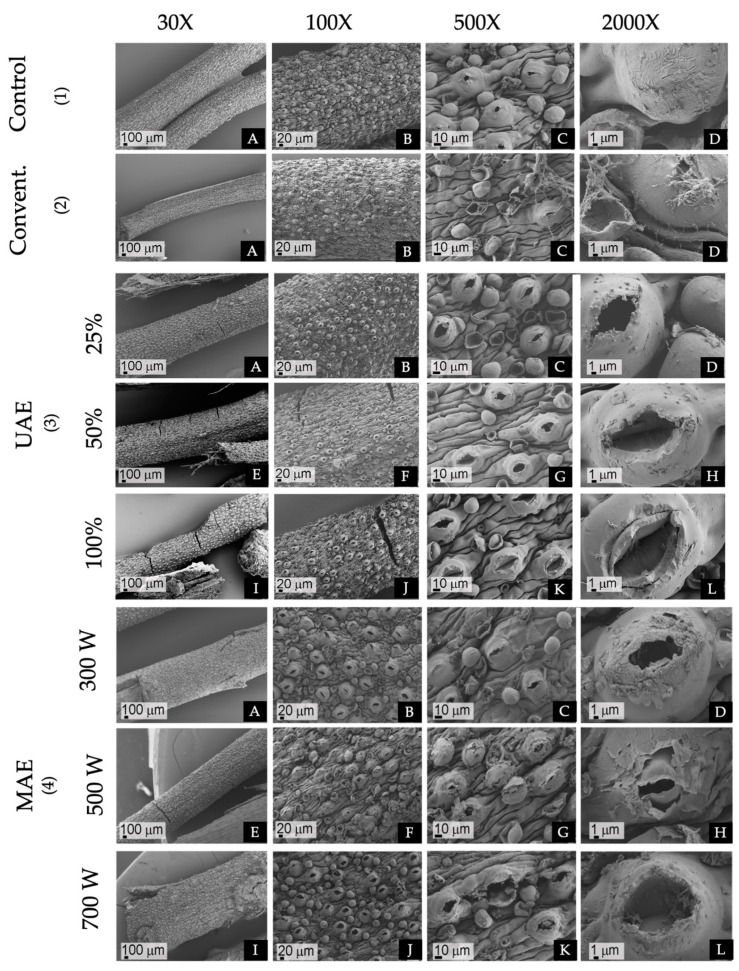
SEM of the material of *F. punensis*. (1)(A–D) Rehydrated in physiological solution, (2)(A–D) Exposed to conventional maceration (Convent), (3)(A–D) Exposed to UAE (ultrasound-assisted extraction) 25% of amplitude, (E–H) 50% of amplitude and (I–L) 100% of amplitude, (4)(A–D) exposed to MAE (microwave assisted extraction) at 300 W of power, (E–H) 500W of power and (I–L) 700 W of power. Capture taken at 30×, 100×, 500× and 2000×.
